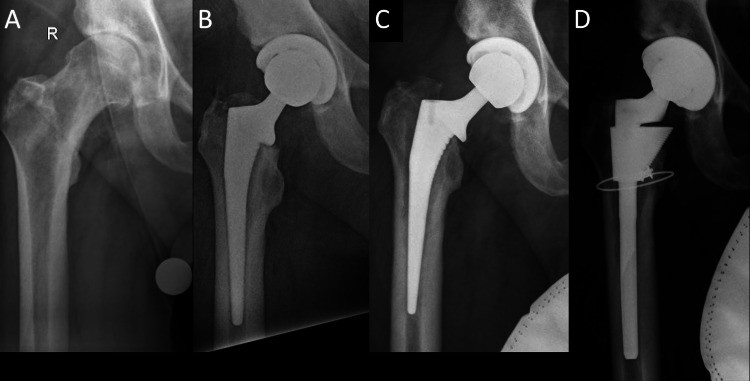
Radiographs of patient 1. (A) Preoperative AP of patient 1 with Dorr A bone and canal-flare index of 5.8. (B) Postoperative AP of Corail size 10, collared, high-offset stem with a +5 mm head. (C) Follow-up radiographs with radiolucent lines in zones 1 and 7 with varus migration. (D) Revision of S-ROM stem. AP, anteroposterior view
